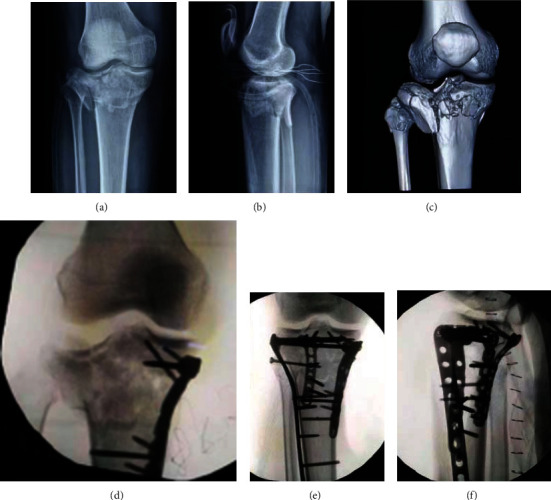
A tibial plateau fracture case combined with a superior tibiofibular dislocation (type 4 injury in four-column nine-segment classification) was fixed by plates and a positioning screw: (a) preoperative A-P view X-ray; (b) preoperative lateral-view X-ray; (c) preoperative three-dimensional CT imaging; (d) intraoperative A-P view X-ray showed the obvious dislocation of the superior tibiofibular joint; (e) intraoperative A-P view X-ray after the placement of the positioning screw; (f) intraoperative lateral-view X-ray.
